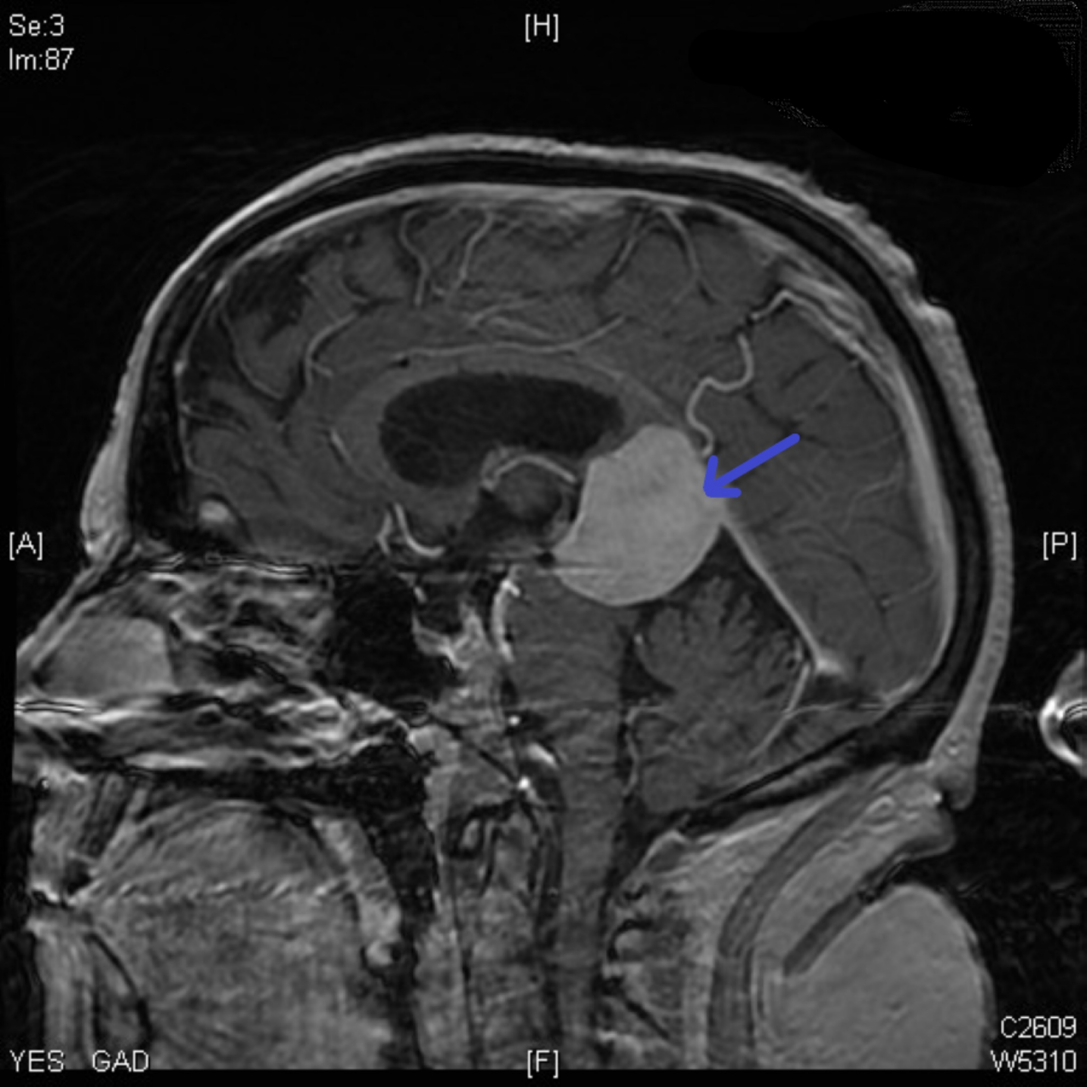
Preoperative MRI displaying the falcotentorial meningioma MRI: magnetic resonance imaging
